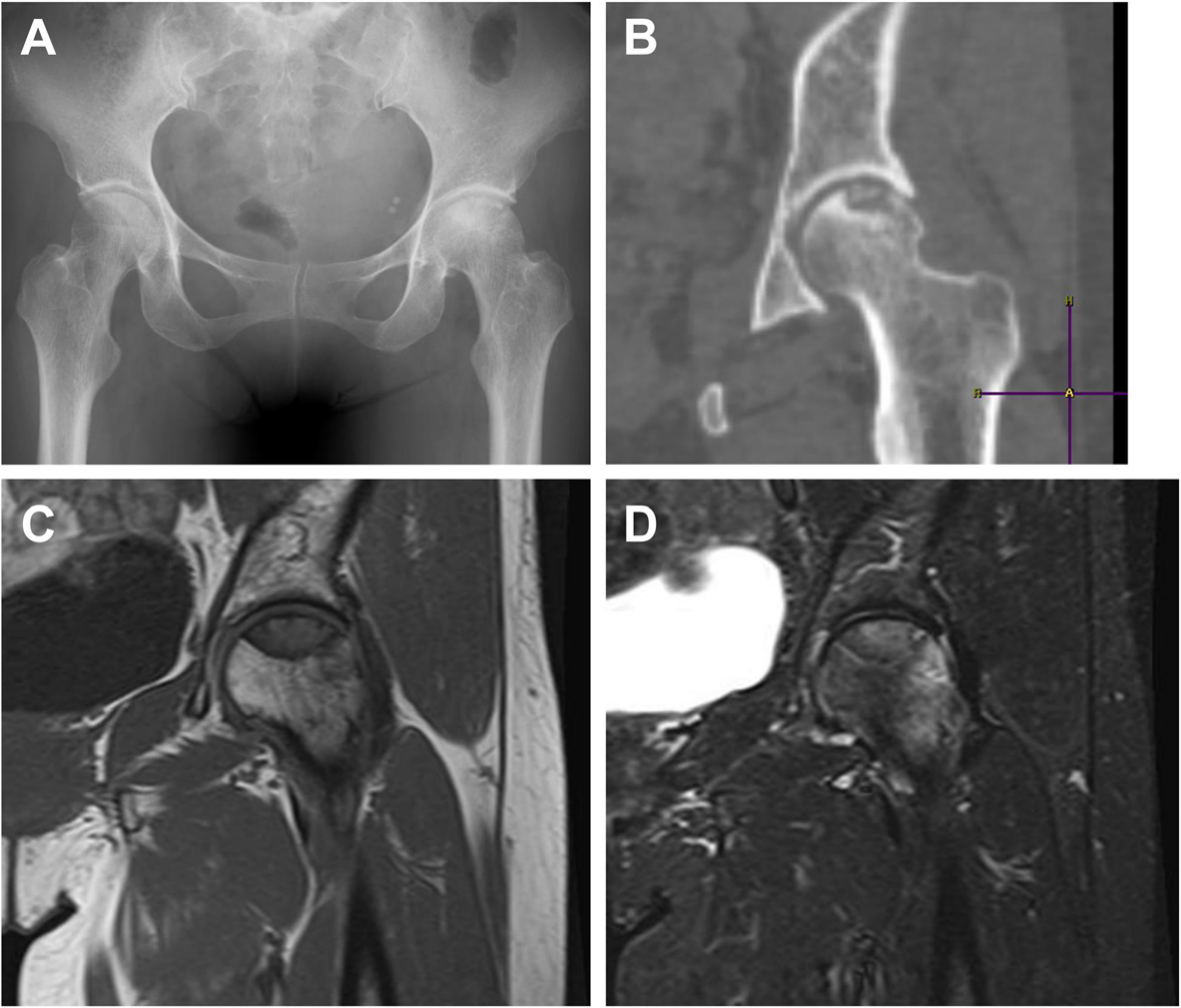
a X-ray of the hip joint showing left partial femoral head collapse. b CT image showed a lesion in the loaded part of the left femoral head. c Coronal T1 weighted image (T1WI) on MRI, showing low intensity of the left femoral head lesion. d Coronal short tau inversion recovery (STIR) on MRI, showing partially high intensity of the femoral head lesion
